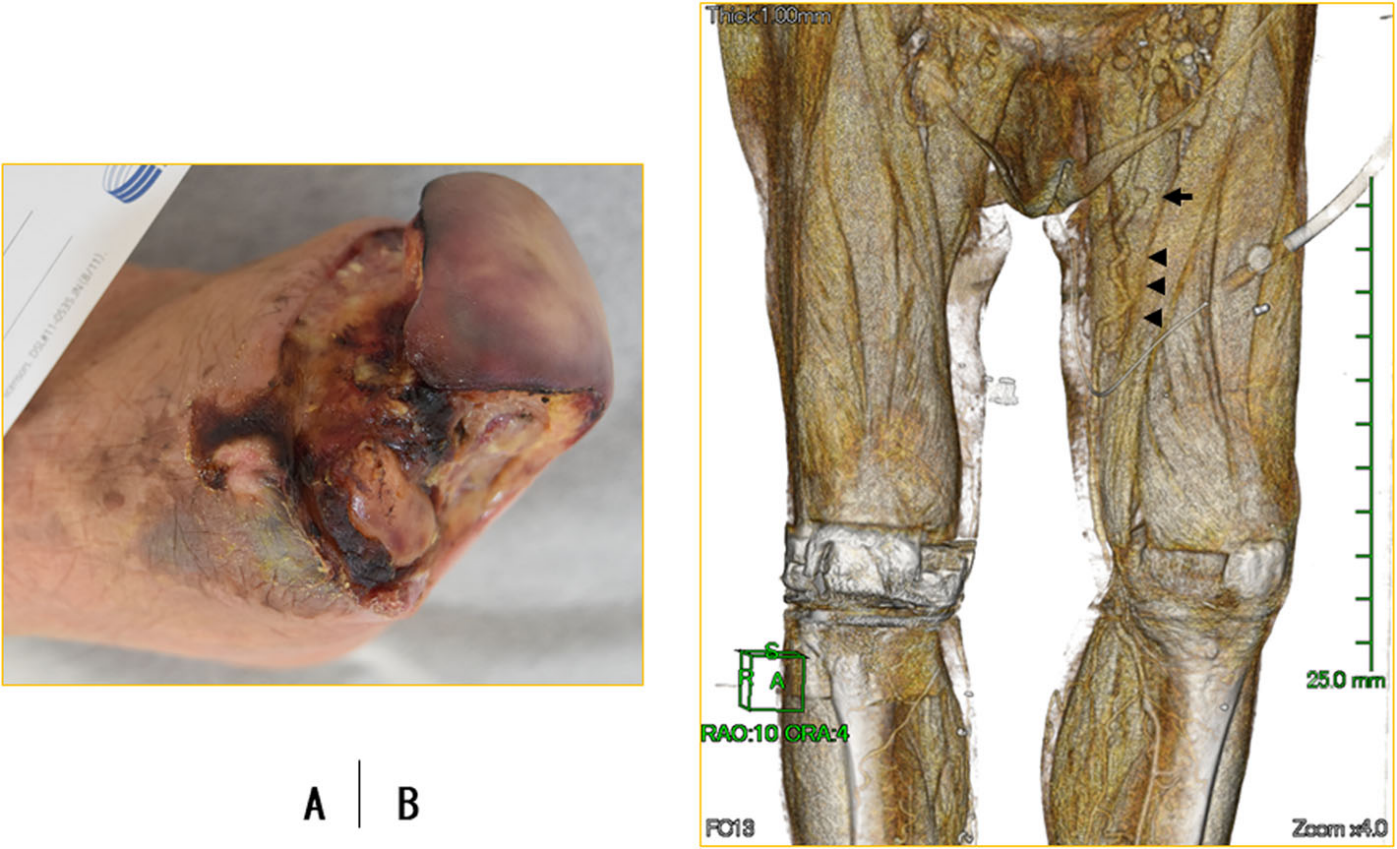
The stump became gradually necrotic after metatarsal amputation (a). Preoperative plain computed tomography showed that the left great saphenous vein was complicated with varicose veins (arrowheads) with thin vessel walls (arrow) (b)
